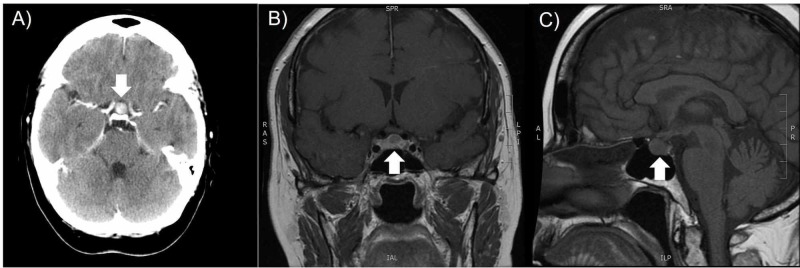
CT and MRI T1-FLAIR images of the pituitary tumor found five years after initial diagnosis and treatment of lung LCNEC. The patient presented with neurological symptoms including unsteady balance, bilateral temporal headaches, memory loss, and mild mood alterations. Images reveal an enhancing pituitary tumor, initially thought to be a pituitary adenoma, measuring 5 mm size with suprasellar extension near the optic chiasm (arrow). (A) CT axial view; (B) MRI T1-FLAIR coronal view; (C) MRI T1-FLAIR sagittal view. LCNEC: Large cell neuroendocrine carcinomas
